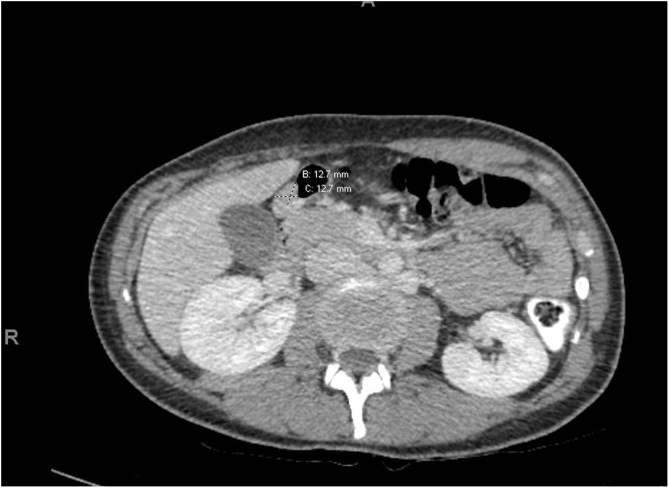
Abdominal CT scan showing the pre-pyloric, homogenously enhancing mass. The lesion has similar appearance as the nearby normal pancreatic tissue.
